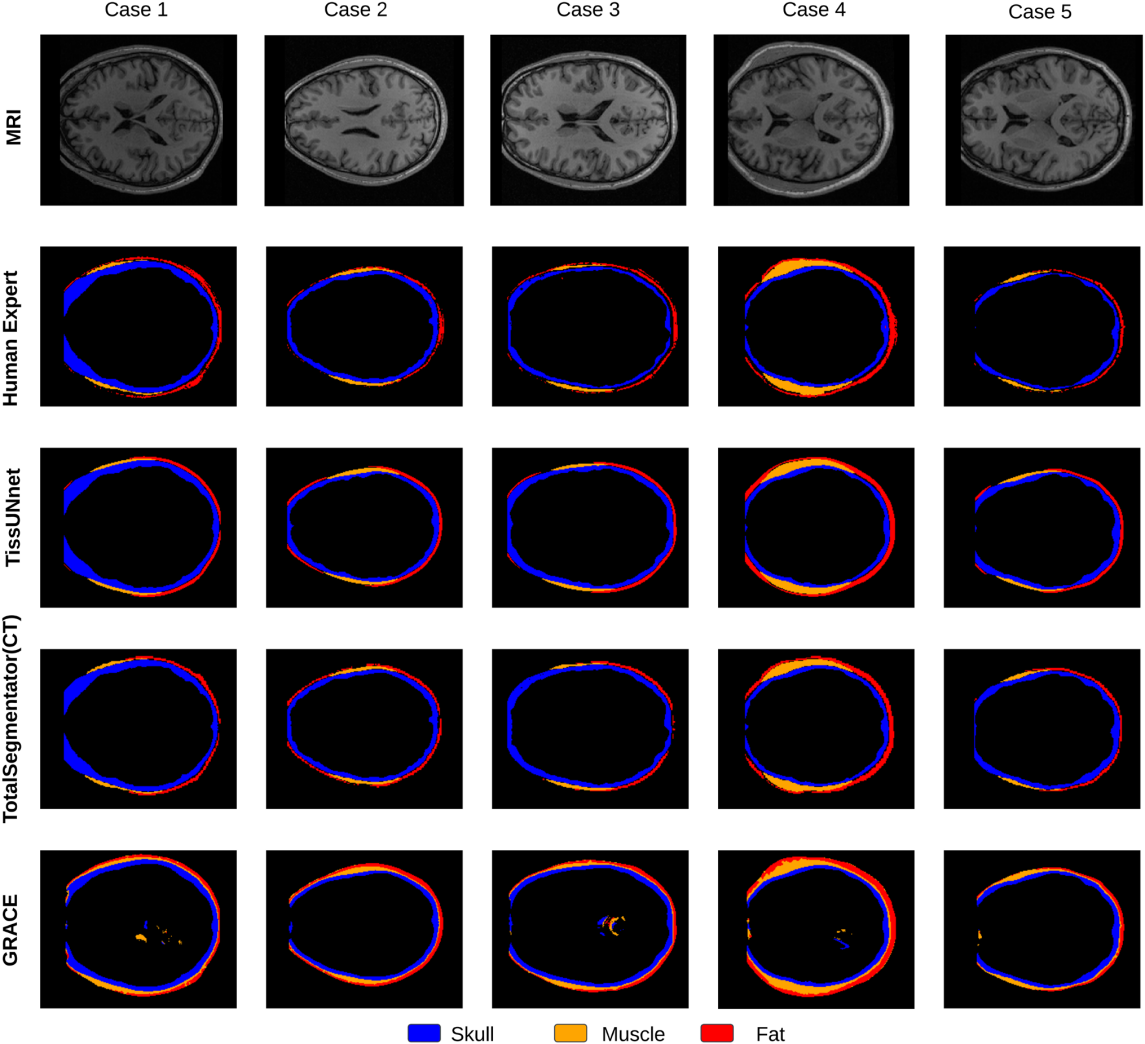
Sample of five cases segmentations for three classes (skull in blue, muscle in yellow, and subcutaneous fat in red) from the T1-MRI. The results are shown in axial view, slice z* = *100. We compared GRACE (MRI T1w) to TissUnet (our), Human Expert annotations, and TotalSegmentator (predicted over CT image).
